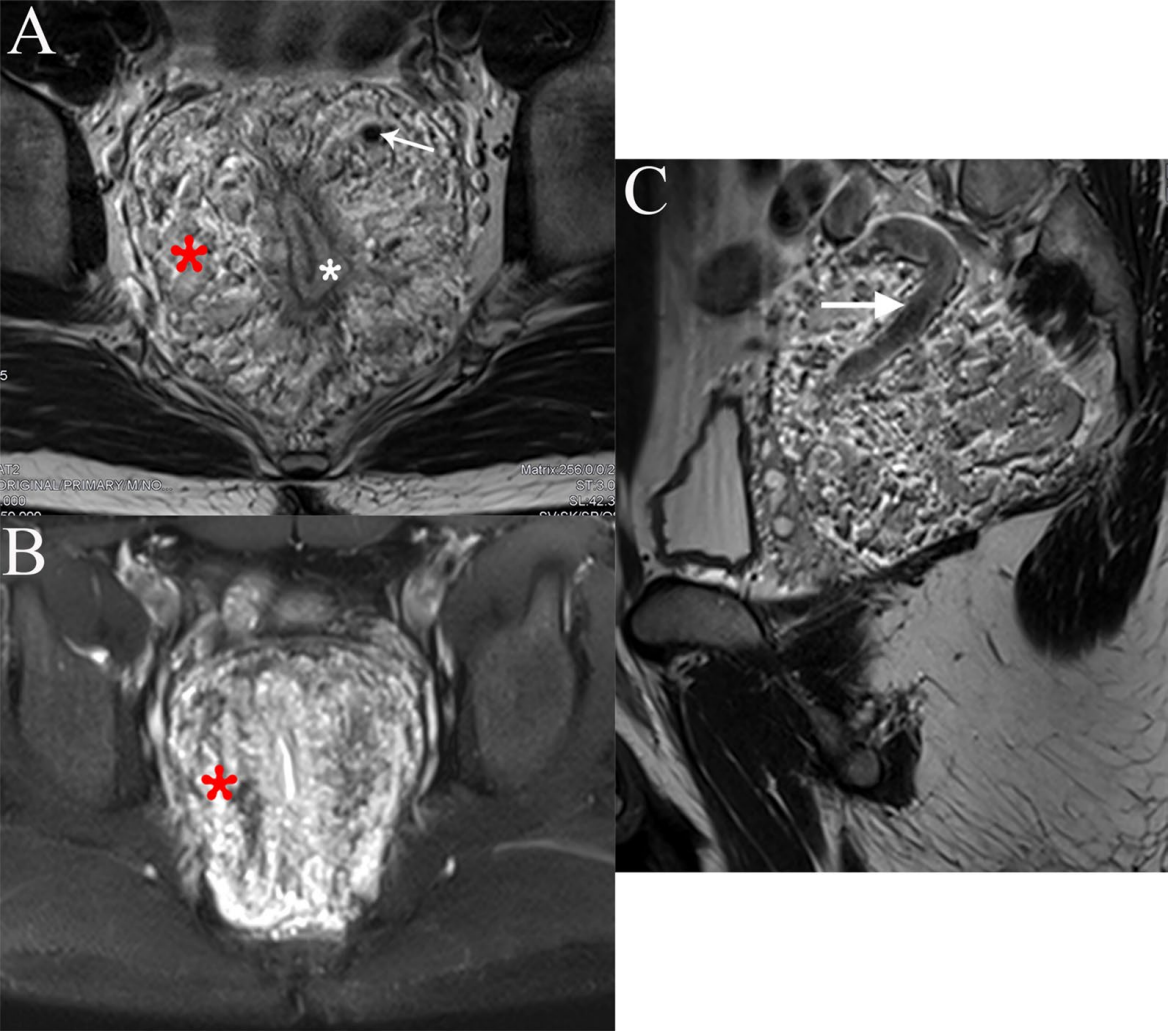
Rectal MRI. A Axial T2-weighted images showing wall thickening of the rectum (white asterisk), heterogeneous high signal intensity of the lesion (red asterisk) and black signal vessel (flowing void effect) (arrow). B Axial fat-suppressed T2-weighted images with the high signal intensity lesion more clearly depicted (red asterisk). C Sagittal T2-weighted images showing the dilatation inferior mesenteric vein (arrow)
